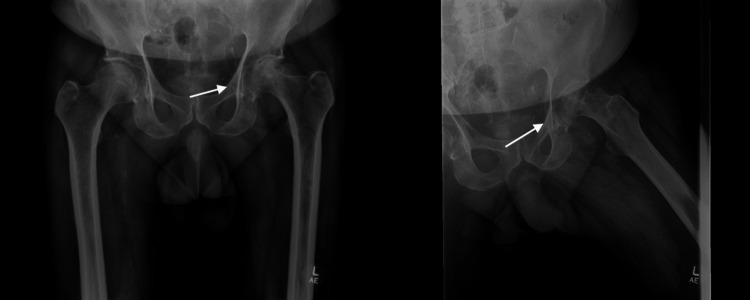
Anteroposterior and lateral X-ray of the pelvis demonstrating significant degenerative changes with collapse of the femoral head
